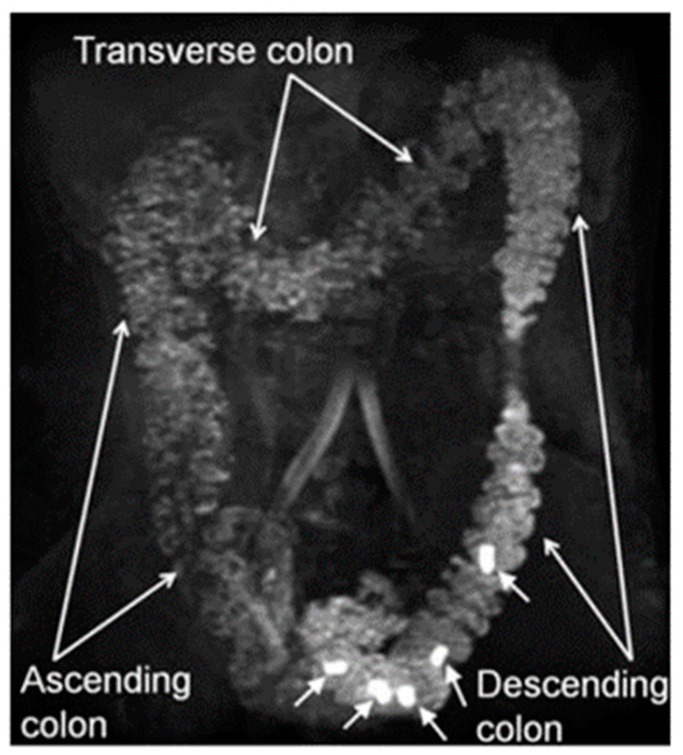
Magnetic resonance imaging (MRI) showing five MRI marker capsules in the colon (indicated by close arrows). Reproduced with permission from [71]; published by John Wiley & Sons, 1994.
